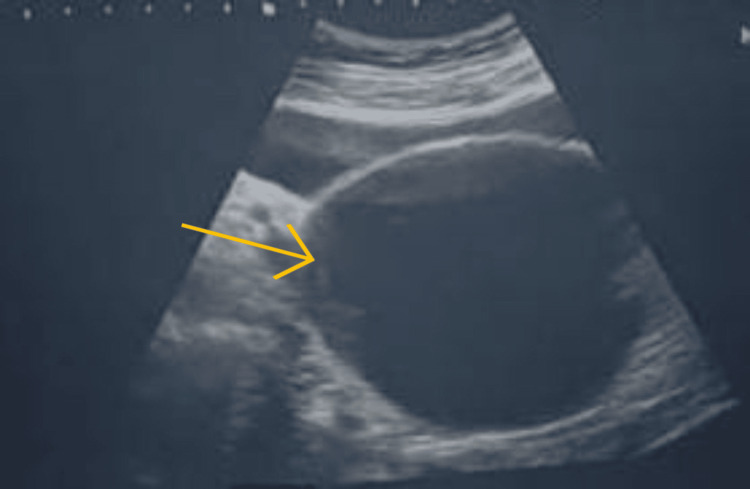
Patient's sonography report indicating hemorrhagic cystic lesion in between two uterine fundus
